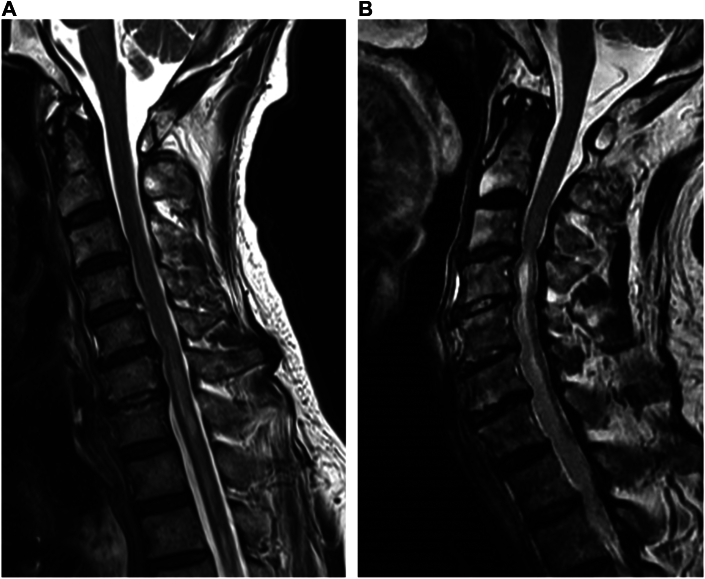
Sagittal T2-weighted magnetic resonance images show spinal cord compression at (A) C5/6 and (B) C4/5 and C5/6 and an increased signal intensity of the spinal cord at (A) C6-T2 and (B) C5-T2.
